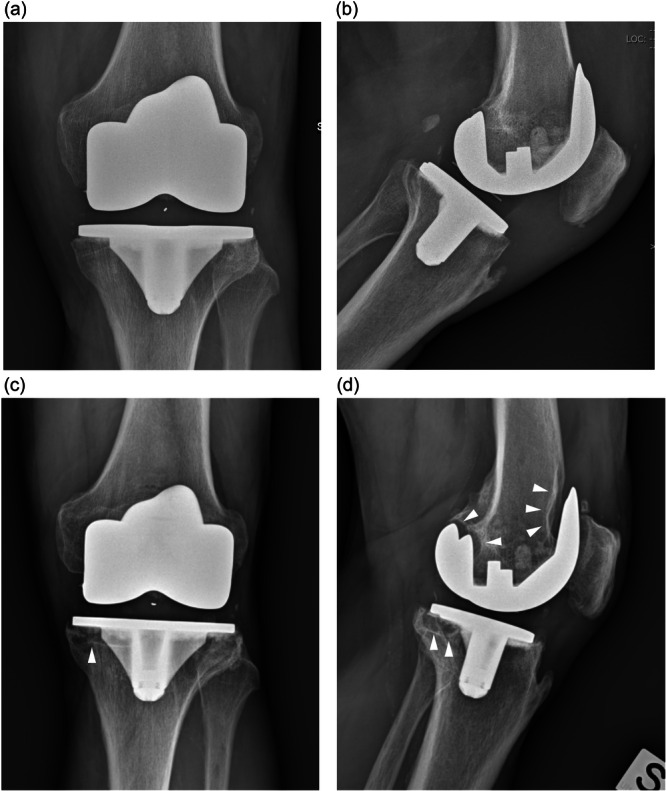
Anterior‐posterior and lateral radiographs of the knee taken immediately postoperatively ((a) and (b), respectively) and at 8‐year follow‐up ((c) and (d), respectively) showing periprosthetic osteolysis (white arrowheads) around knee implant with VEPE tibial insert. VEPE, vitamin E‐stabilized polyethylene.
